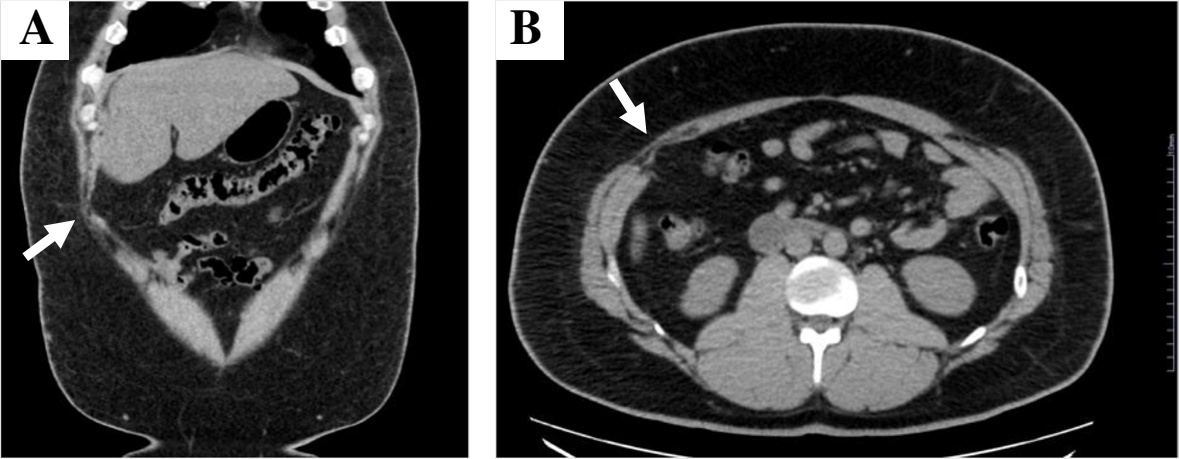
The transverse abdominal muscle shows atrophy at the postoperative scar on plain abdominal coronal computed tomography (a) and plain abdominal transverse computed tomography (b). *Arrows* indicate adhesive region of the greater omentum
